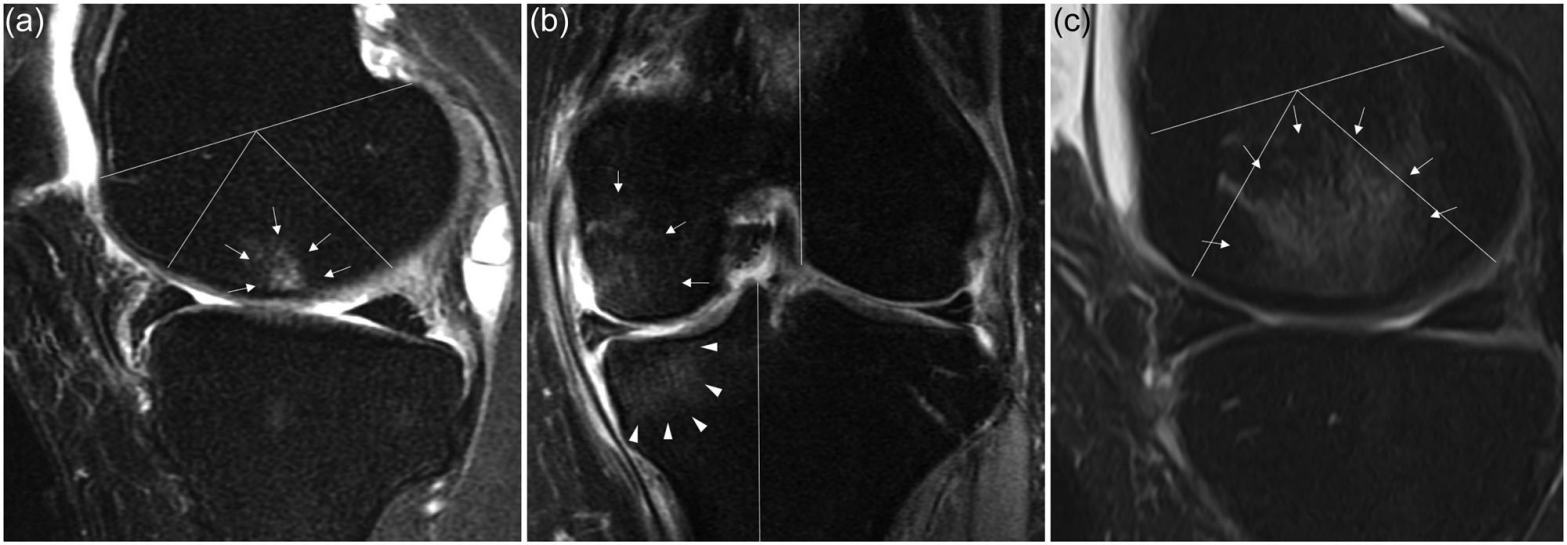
BME severity assessment based on the extent of its anatomically defined subregional involvement observed on MRI. (a) Sagittal T2 fat suppression image with Grade 1 BME depicted on the central subregion of medial femur (arrow). (b) Coronal T2 fat suppression image with Grade 2 BME depicted on medial tibia subregion (arrowhead) and Grade 1 BME on central subregion of medial femur (arrow). (c) Sagittal T2 fat suppression image with Grade 3 BME depicted on central subregion of medial femur. BME, bone marrow oedema; MRI, magnetic resonance imaging.
